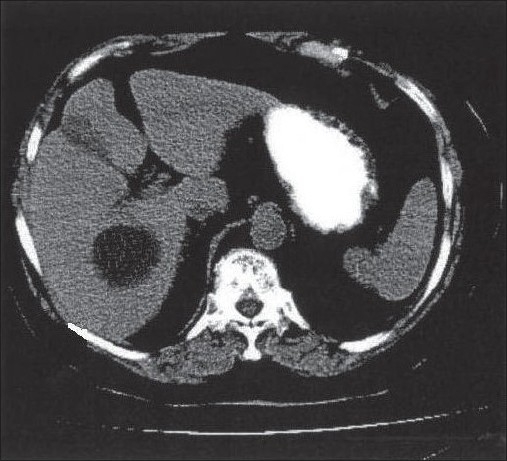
Original Abdomen CT Image
